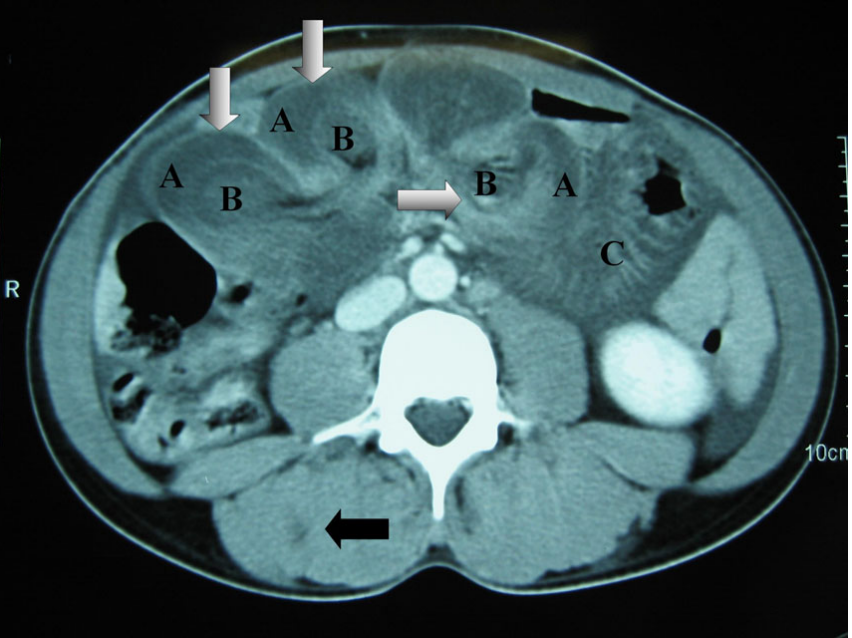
CT scan of abdomen showing i) multiple intussusceptions demonstrating doughnut signs (white arrows), intussusceptiens [A], intussusceptum [B], distended loop of small bowel [C] and ii) haemangioma of right quadratus lumborum muscle (dark arrow).
